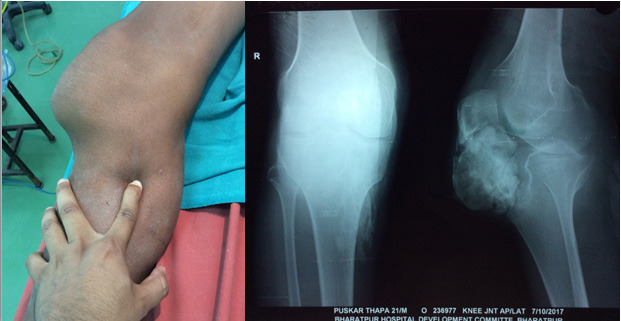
Preoperative clinical picture and radiograph.
